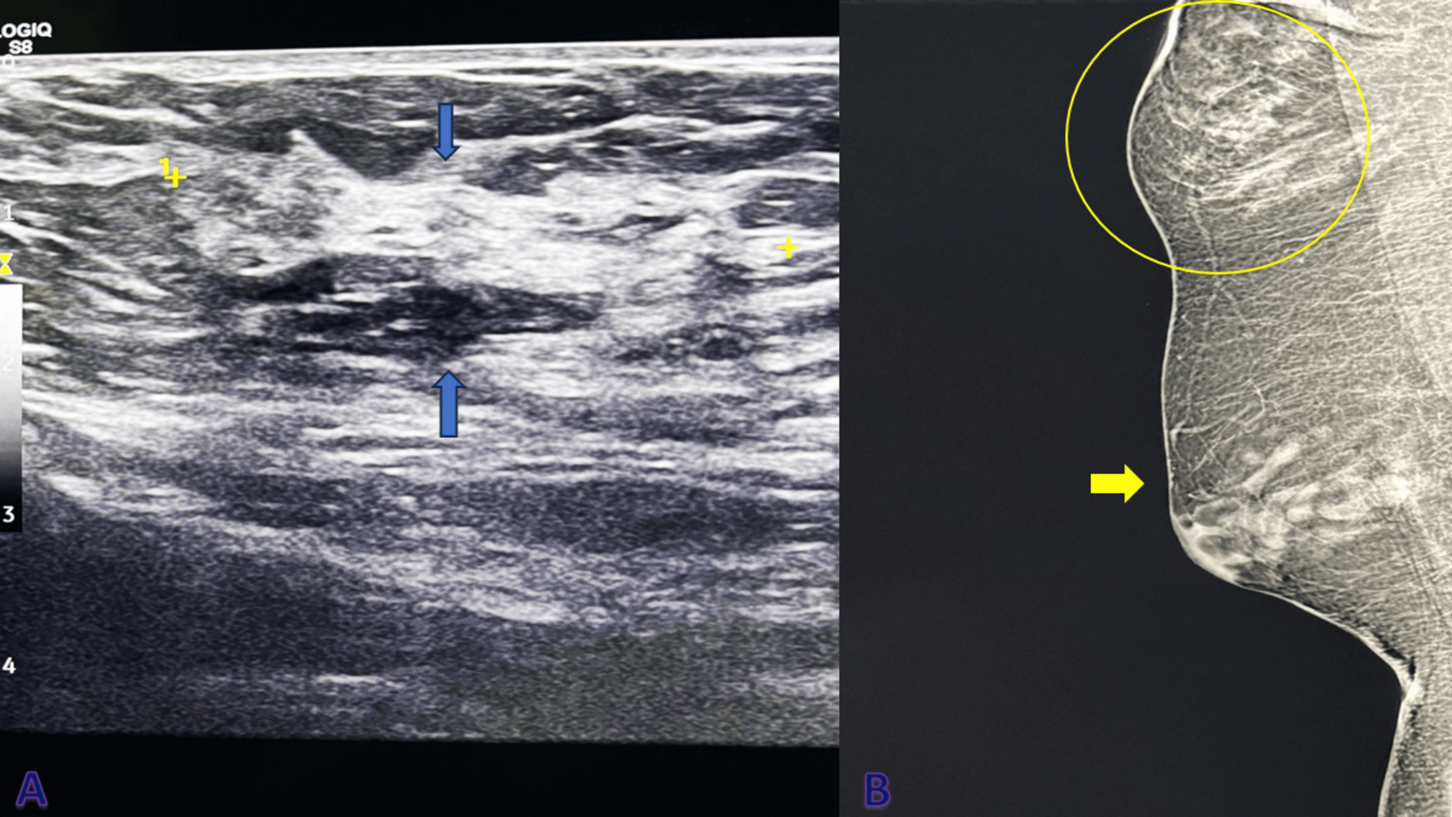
Α. Axillary ultrasound showing a small hypoechoic superficially located mass with irregular margins measuring 3.41 cm (in length which is indicated between the yellow crosses) in the right axilla resembling the features of fibrograndular breast tissue (blue arrows). Β. Appearance of accessory breast tissue on the mammography posterior plane on a mediolateral oblique view of a 19-year-old woman. Asymmetry (circle) is noted on the right, upper hemisphere due to the presence of a lipomatous breast-resembling mass outside the normal breast tissue (yellow arrow).
